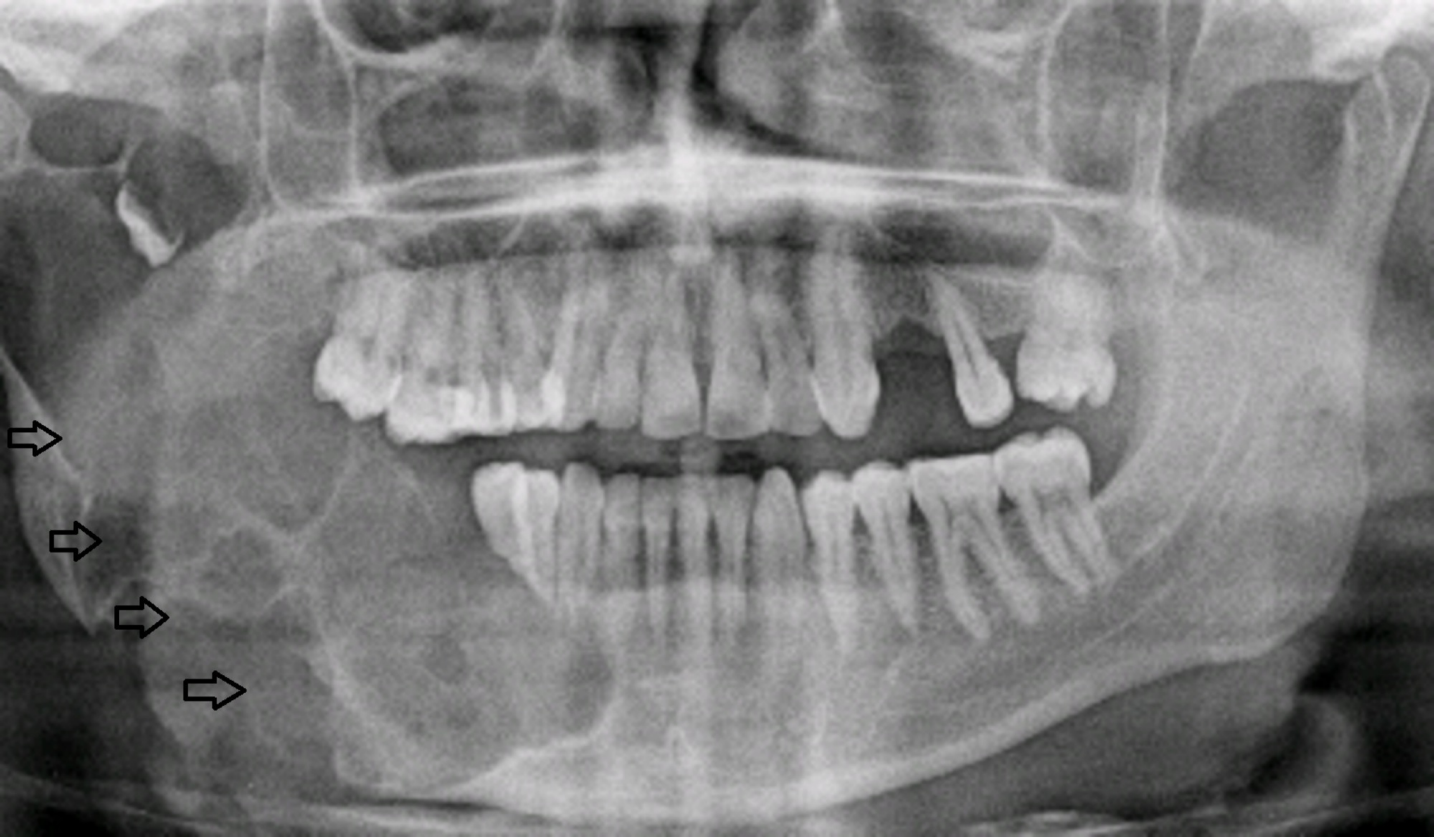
Patient 14. A 38-year-old male with right-sided facial swelling. Preoperative orthopantomogram revealed multilocular lucencies (arrows) on the right side.
